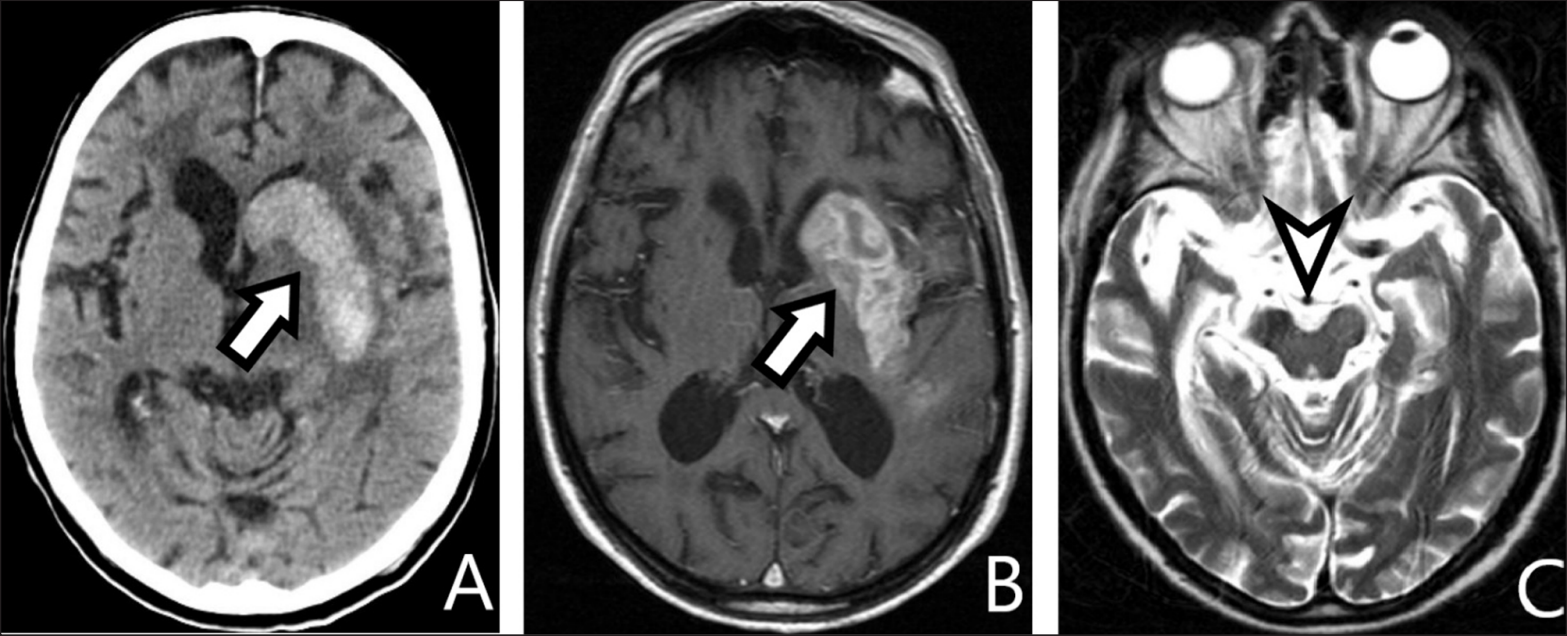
A: *axial soft-tissue window CT*, B: *T1-Gd*, C: *T2-WI*. Left intracerebral hemorrhage in the caudate and lentiform nuclei, hyperdense on CT and hyperintense on MRI (*arrows*). The cerebral peduncles symmetric (*arrowhead*).
